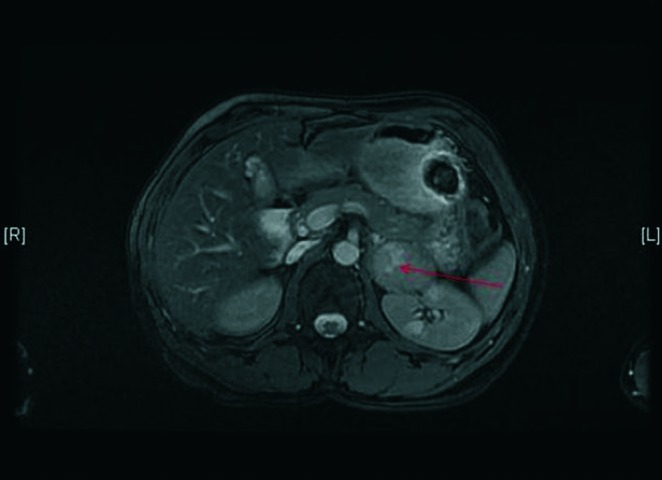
Sagittal T2-weighted MRI of abdomen showing left adrenal mass. (indicated by arrow)
